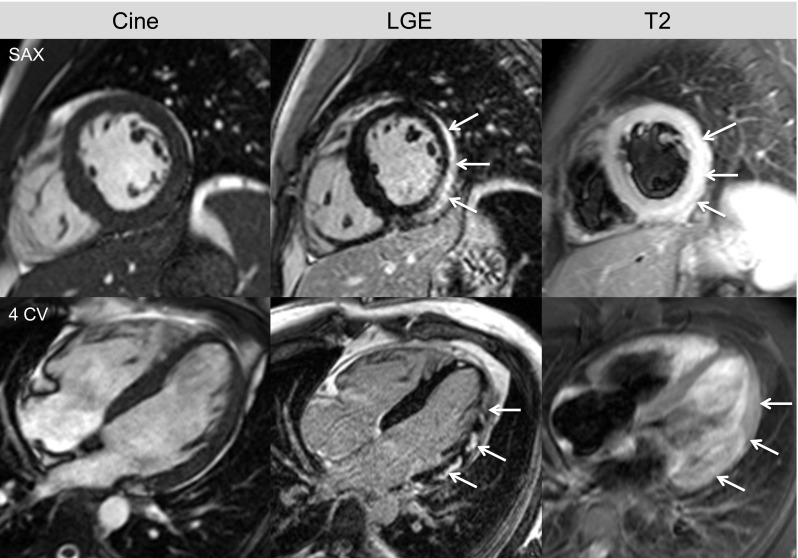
CMR of a 28-year-old male presenting with dyspnea and chest pain 5 days after flu-like symptoms. Due to ST elevation and elevated cardiac enzymes, the patient underwent coronary angiography where coronary stenosis could be excluded. Cine images reveal global preserved left ventricular ejection fraction (LVEF = 60 %) with discrete hypokinesia at the lateral wall. LGE is present in multiple locations (*white arrows*), suggestive of myocarditis. The acute nature of the disease is demonstrated by positive dark-blood T2W images displaying a clear bright signal in the lateral wall, in an area consistent with LGE findings. Note that on the correspondent T2-weighted images in the long-axis, these lesions are not well delineated. This patient received state of the art heart failure medication (betablocker, ARB); in the next few months, the initial extensive LGE lesions decreased in size (which could be observed at a subsequent CMR scan) and the patients complaints resolved completely
